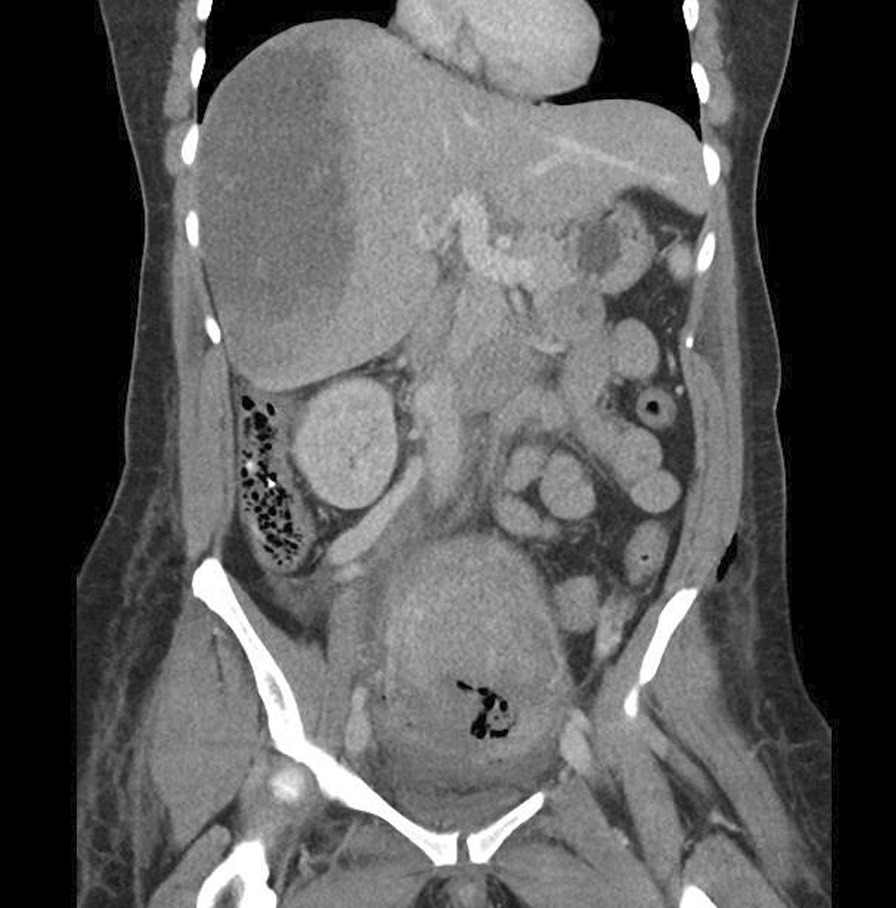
1. Initial CT abdomen: Large subcapsular liver hematoma measuring 16 cm in maximum cranial-caudal dimension. Heterogeneous attenuation is compatible with blood product of varying ages. No active bleed was identified. There is significant mass effect on the liver, however the liver parenchyma was normal.
